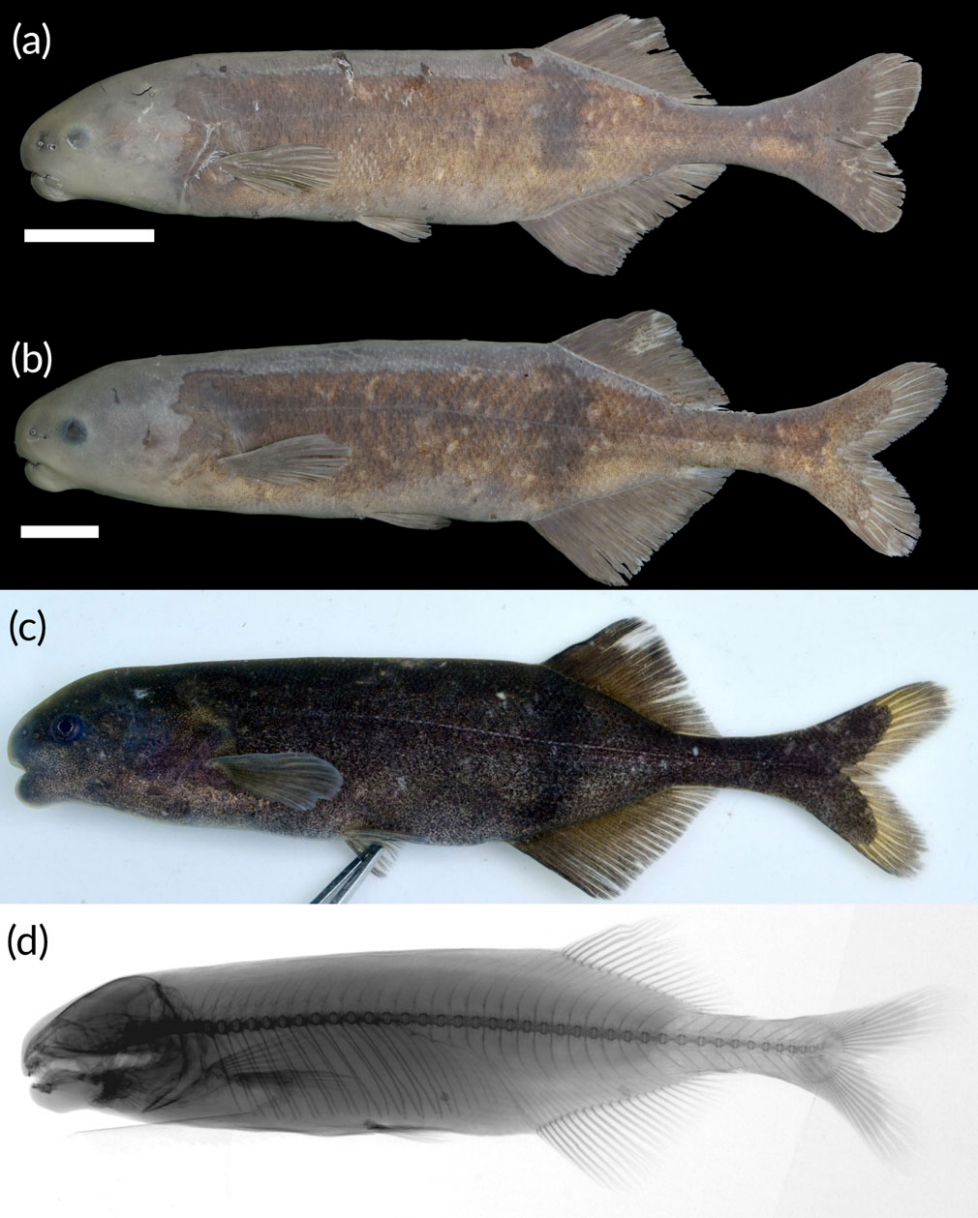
(a) Holotype of *Heteromormyrus xanekweorum* sp. nov. (SAIAB 186792), male 66.7 mm standard length (SL), from the Okavango River, southern Africa; (b) paratype of *H. xanekweorum* sp. nov. (SAIAB 186792), female 99 mm SL, from the Okavango River, southern Africa; (c) live picture of *H. xanekweorum* sp. nov.; (d) X‐ray radiograph of holotype. Scale bar =1 cm.
